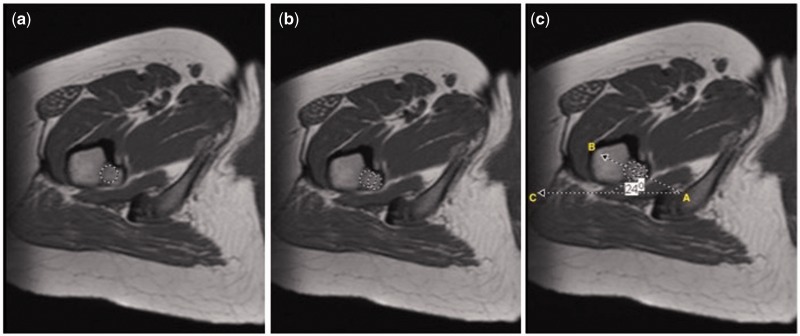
Axial T1-weighted MR images demonstrating a two-centroid method to measure lesser trochanteric version: An image demonstrating the largest width of the LT is selected. (a) The first centroid is placed at the midline lesser trochanteric base; (b) The second centroid is placed at the border of the lesser trochanteric tip; (c) Axis of LT represented by the line AB; the horizontal line is represented by the line AC. The angle CAB forms the LT axis.
